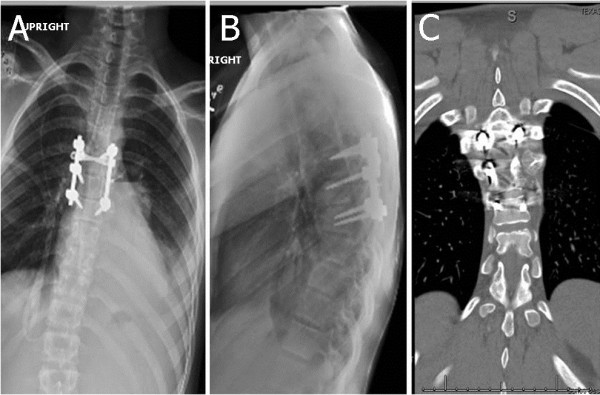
Follow-up (A) anterior-posterior and (B) lateral X-rays, and (C) a coronal computed tomography (CT) scan at three months after surgery shows no evidence of recurrent or residual tumor. There is evidence of bony healing without signs of instrumentation failure.
